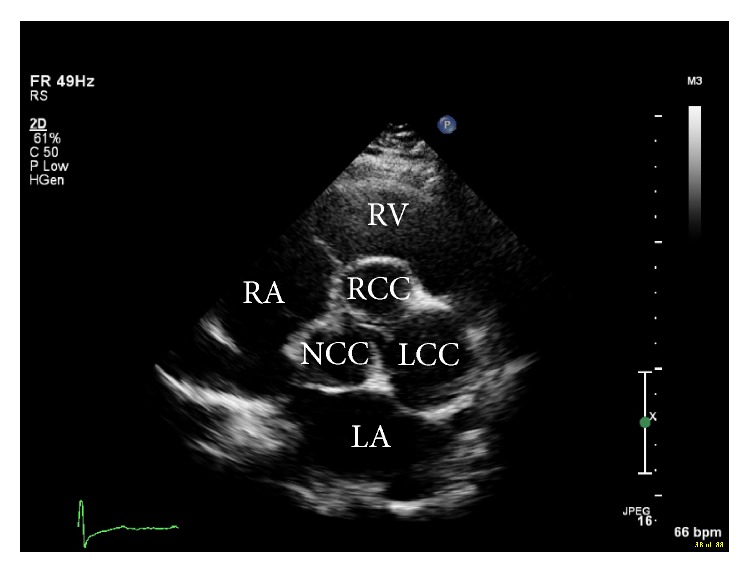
Parasternal short axis view of the aortic valve shows dilated left sinus of Valsalva. Note that the left coronary cusp (LCC) is markedly enlarged compared to the other two cusps. Normally, all three cusps are equal in size. RV: right ventricle, RA: right atrium, and LA: left atrium. RCC: right coronary cusp; NCC: noncoronary cusp.
